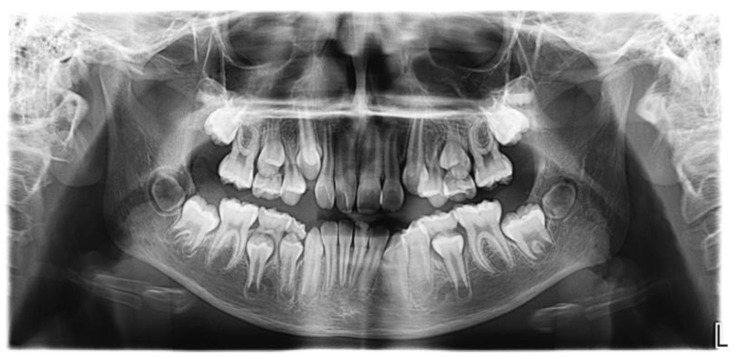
Panoramic X-ray. 2020, four years after the first surgery. Maxillary sinuses of ample development, shape, contour, and characteristic transparency. Stage II mixed dentition. 13, 43, and 33 lack radiographic space for a correct position in the dental arch. Third molars in intraosseous evolution.
